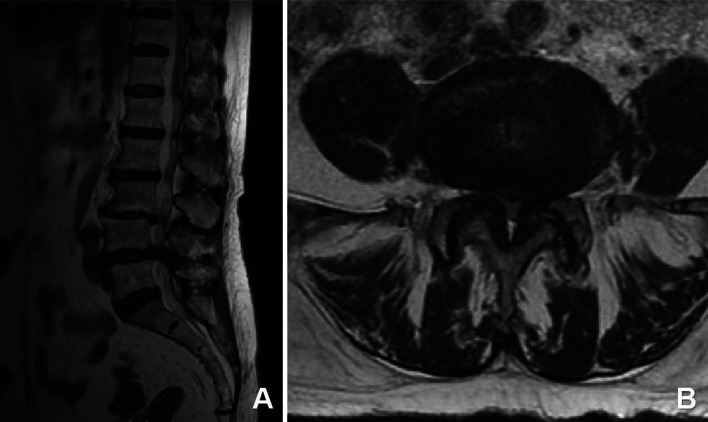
Preoperative magnetic resonance imaging (MRI) examination revealed the presence of spinal stenosis at the L4/5 level.
